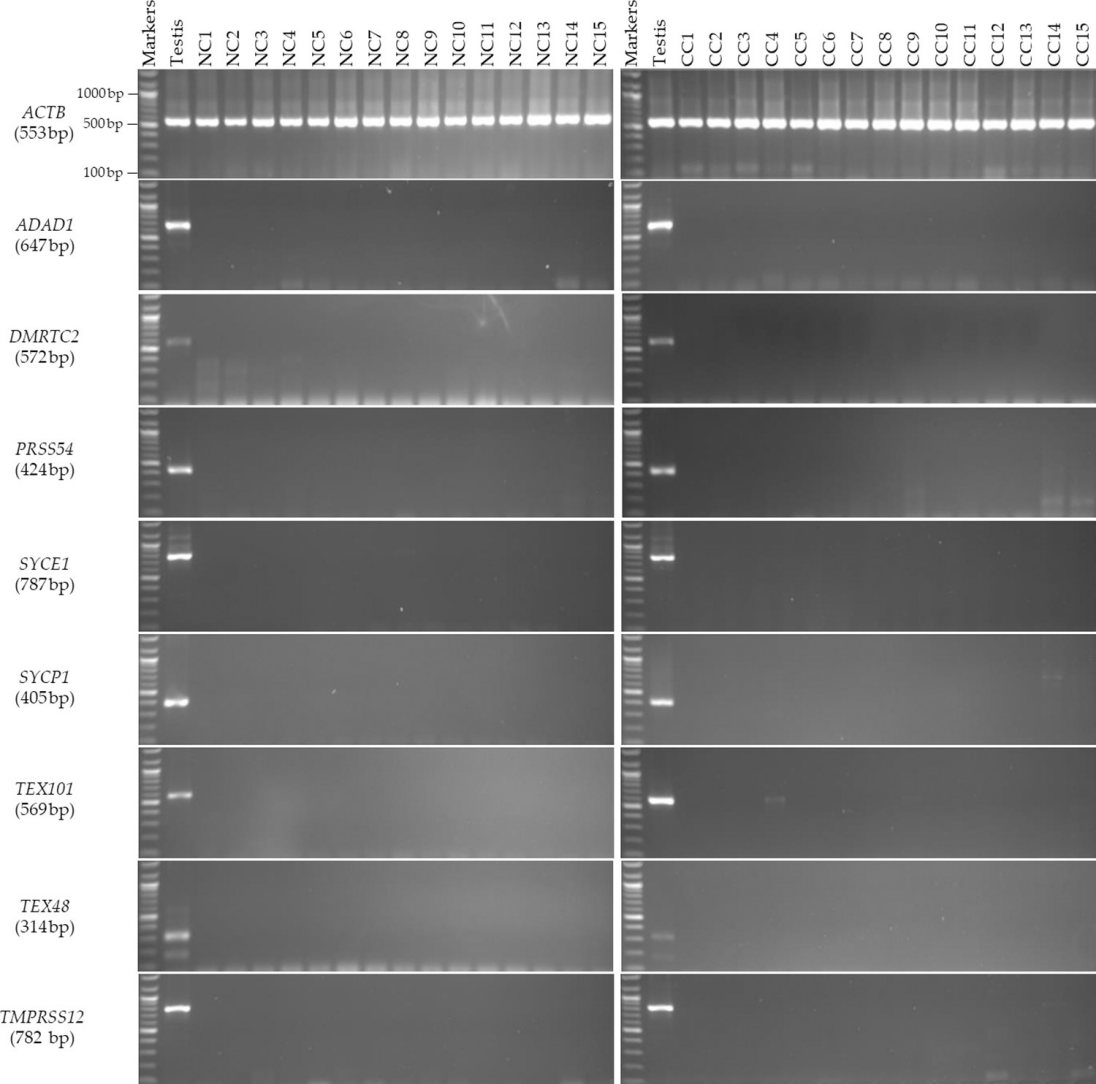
RT-PCR evaluation of the expression patterns of a subset of CT genes in the NC and CC tissue samples from Saudi patients. The results of the RT-PCR analysis of *ADAD1*, *DMRTC2*, *PRSS54*, *SYCE1*, *SYCP1*, *TEX101*, *TEX48*, and *TMPRSS12* are displayed in the agarose gel images. Each cDNA sample was obtained by first isolating total RNA from 15 CC tissue samples and 15 neighboring NC tissue samples. Human *ACTB* expression was used to evaluate the efficacy of each cDNA sample. Human testis cDNA was used to assess the primer quality of each gene. The official symbol and anticipated product size of each studied gene are displayed on the right side of the agarose figure.
